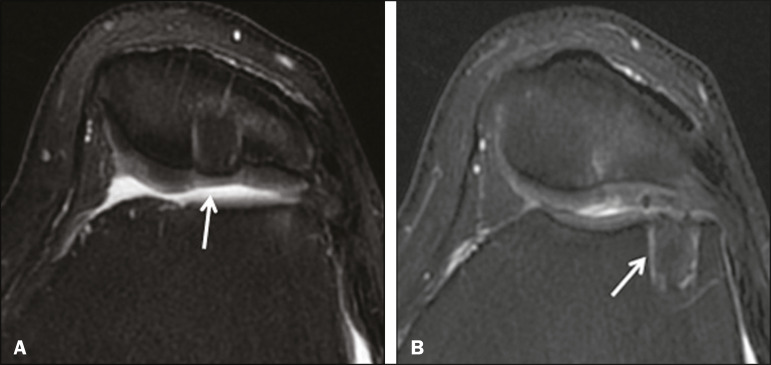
A 39-year-old man who underwent arthroscopic mosaicplasty on the lateral aspect of the patella, with a favorable evolution. Axial T2-weighted fat-saturated MRI sequences, obtained one year after the procedure, demonstrate a nearly completely filled defect, with only a small central incongruity on the articular surface (arrow in A) and minimal osteochondral irregularity at the graft collection site on the lateral aspect of the femoral trochlea (arrow in B).
